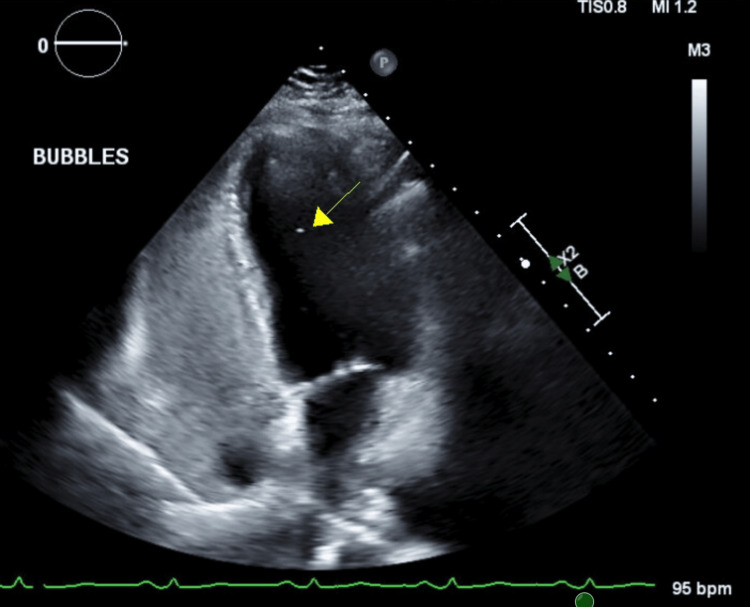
TTE With Bubble Study Normal TTE with a possible patent foramen ovale with a bubble present in the left ventricle, shown by the yellow arrow. TTE: Transthoracic Echocardiogram
